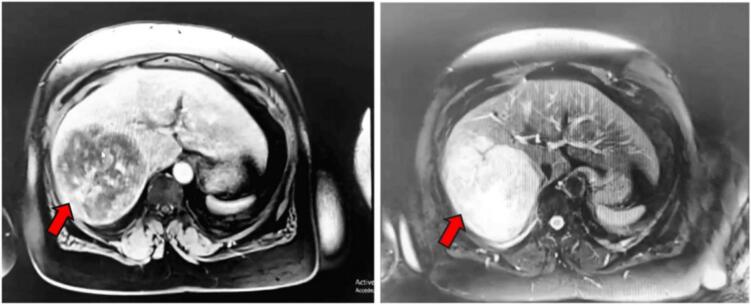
Hepatic MRI image showing the mass occupying segments 6 and 7 indicated by the red arrow. (For interpretation of the references to colour in this figure legend, the reader is referred to the web version of this article.)
